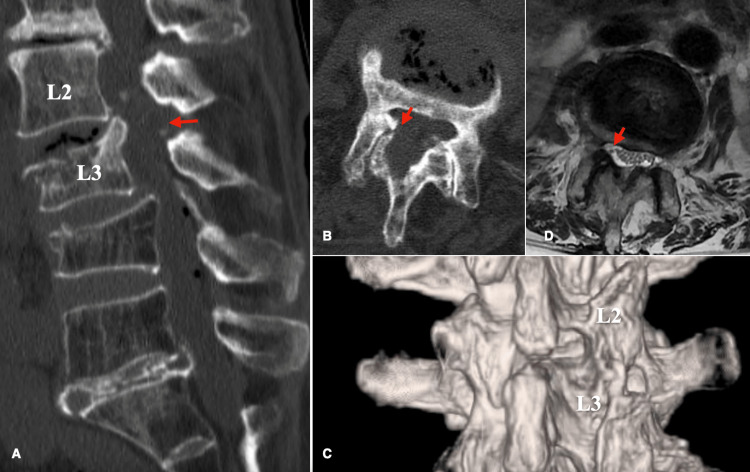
Postoperative imaging. (A) Sagittal CT confirmed that the osteophytes had been resected (arrow). (B) Axial CT confirmed the osteophytes extending from the superior articular process were resected (arrow). (C) 3DCT confirmed that the inter-laminar space was decompressed while preserving the facets. (D) Axial MRI confirmed that the dural mater was decompressed (arrow). 3DCT: 3-dimensional computer tomography.
